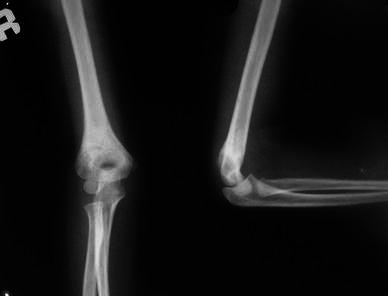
Final follow-up radiograph at 1 year depicting remodeling at the fracture site
